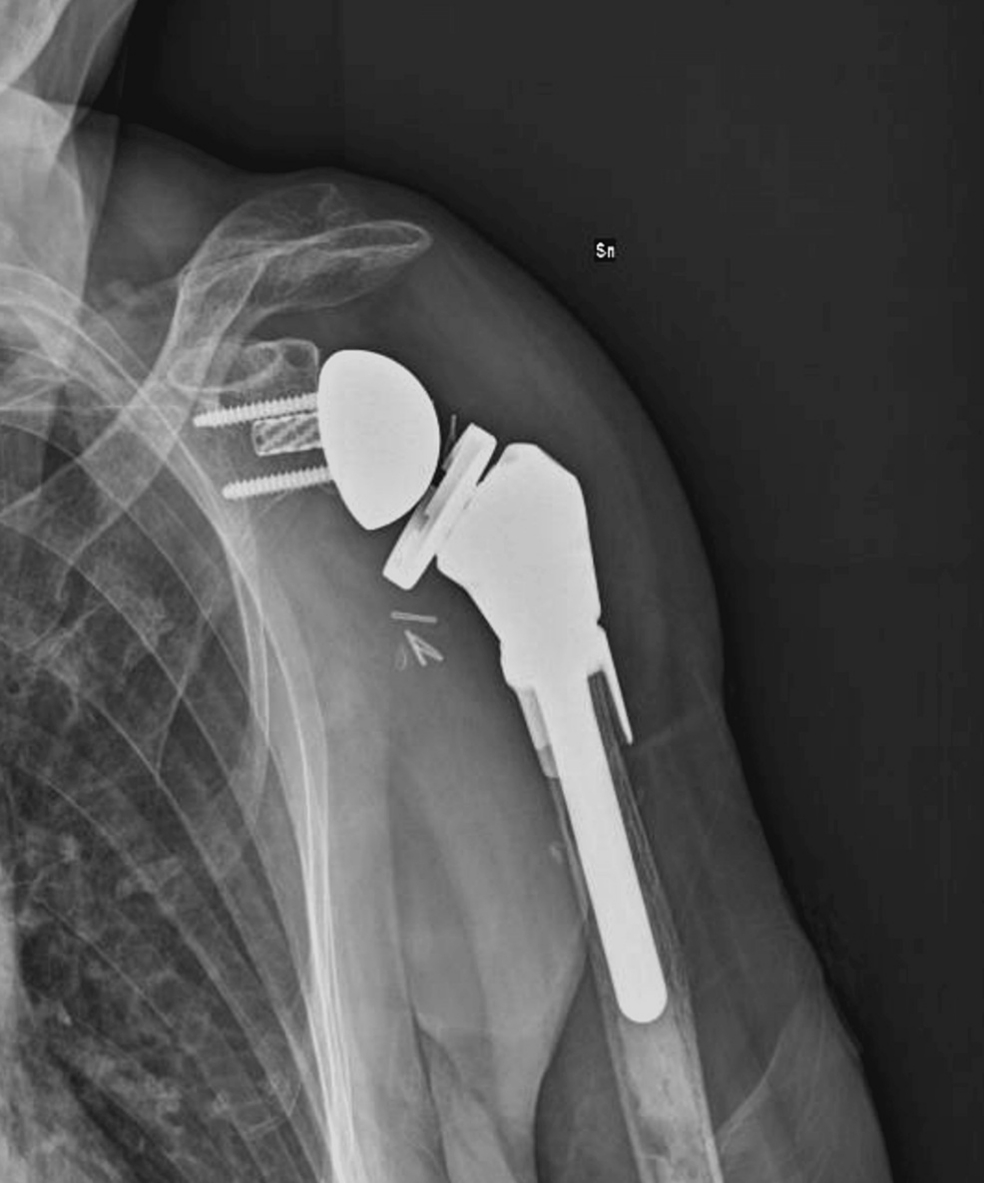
Twelve-month post-megaprosthesis X-ray evaluation (Case 1) Sn: left
